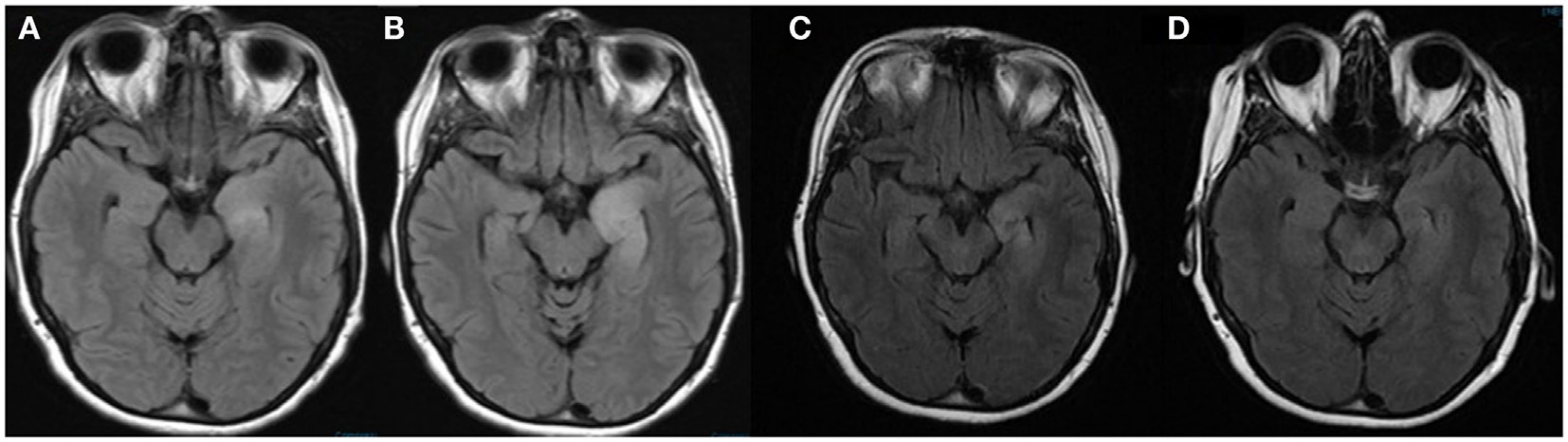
MRI brain FLAIR sequences performed on admission to our hospital (A), 7 days after admission (B), 1 month after discharge (C), and 4 months after discharge (D). These images demonstrate the evolution of the left mesial temporal lobe lesion during admission and improvement 1 month and 4 months after discharge.
